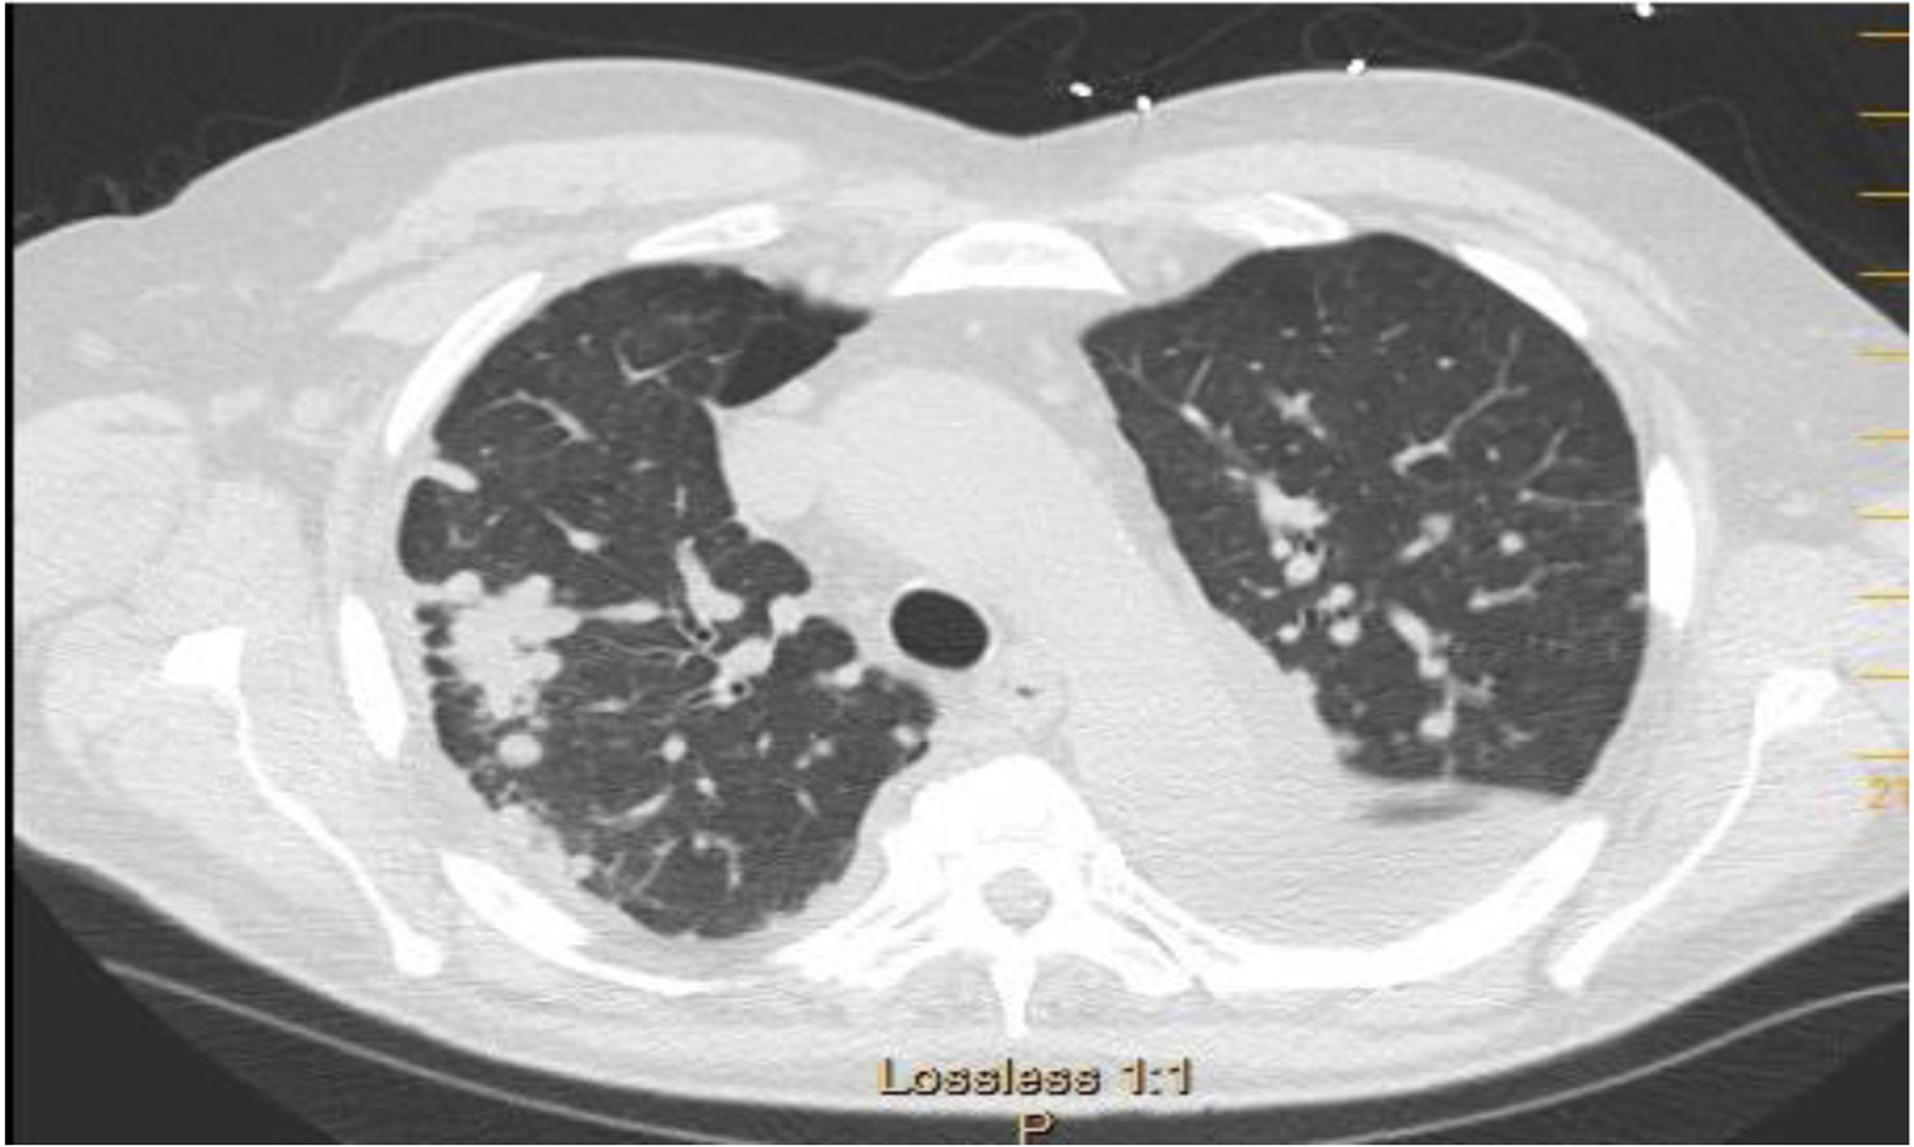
Axial CT chest showing nodules coalescing in to one large conglomerate. Black arrow pointing to coalescing nodules.
